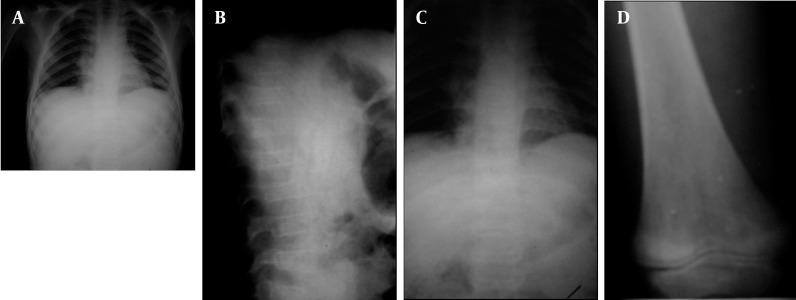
An 8-year-old boy with ALL presenting with anemia, fever, back pain and limping A, CXR reveals perihilar and retrocardiac infiltration. Abnormal shaped vertebra is suspicious.; B and C, Thoracolumbar AP and Lat X-Rays reveal multiple collapsed vertebrae; D, Knee X-xay reveals hypodensity around the knee joint with metaphyseal translucency.
